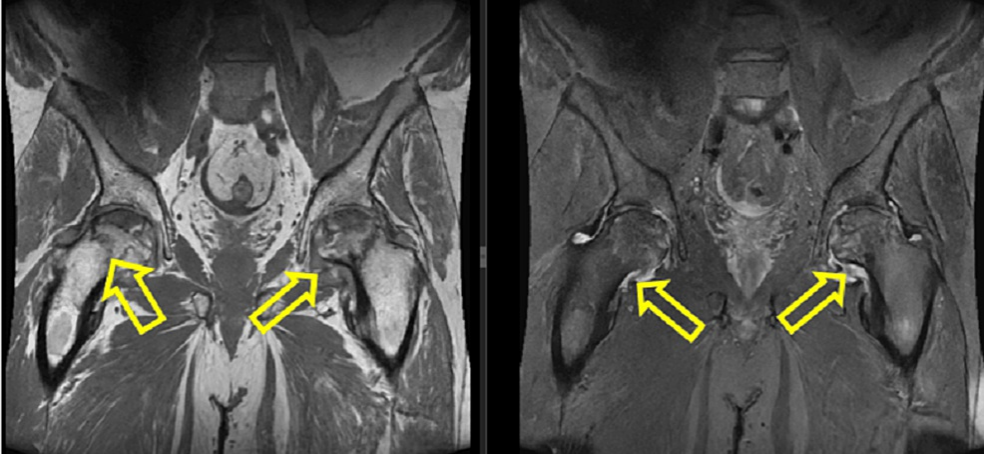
MRI, coronal planes. MRI of the hip joints discovered bilateral avascular necrosis of the hip joints
